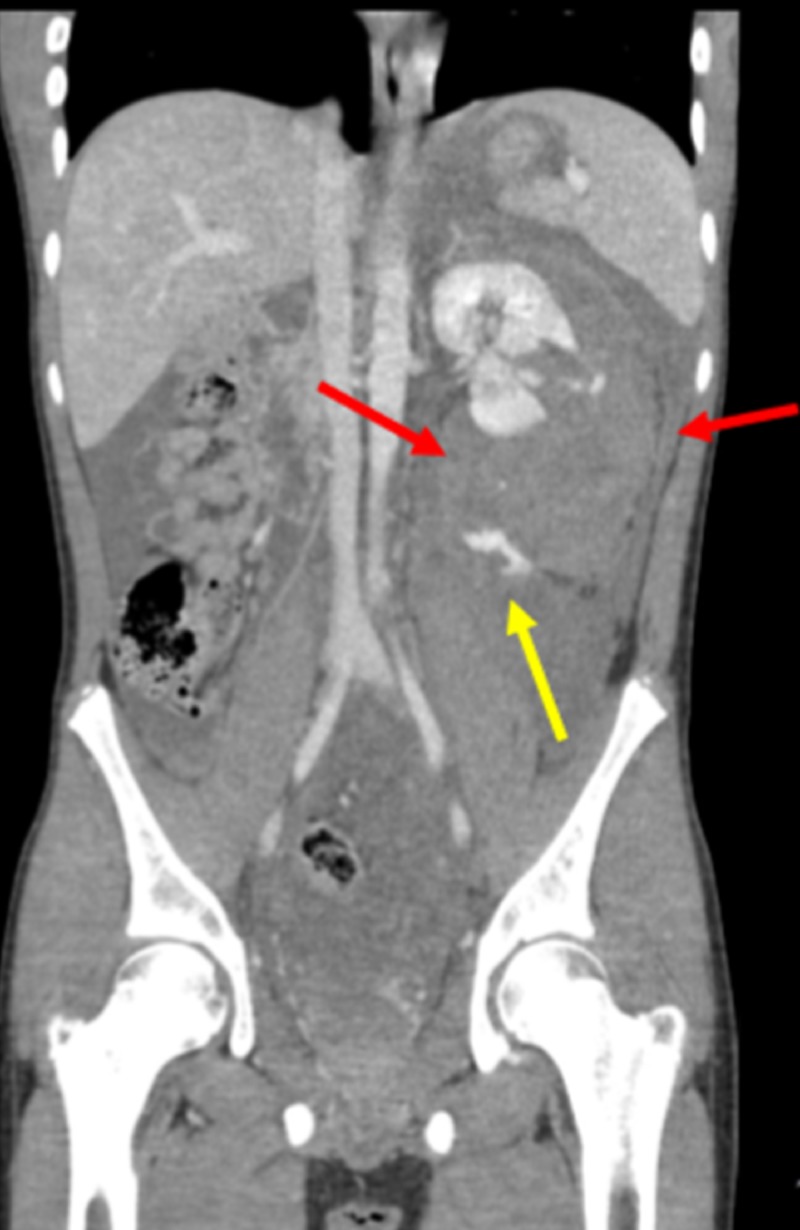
Initial Contrast-enhanced Coronal Computed Tomography of the Abdomen and Pelvis The initial contrast-enhanced coronal computed tomography of the patient shows a perinephric hematoma near the left kidney (borders of the hematoma are shown by the red arrows). The yellow arrow shows active contrast extravasation indicating hemorrhage.
